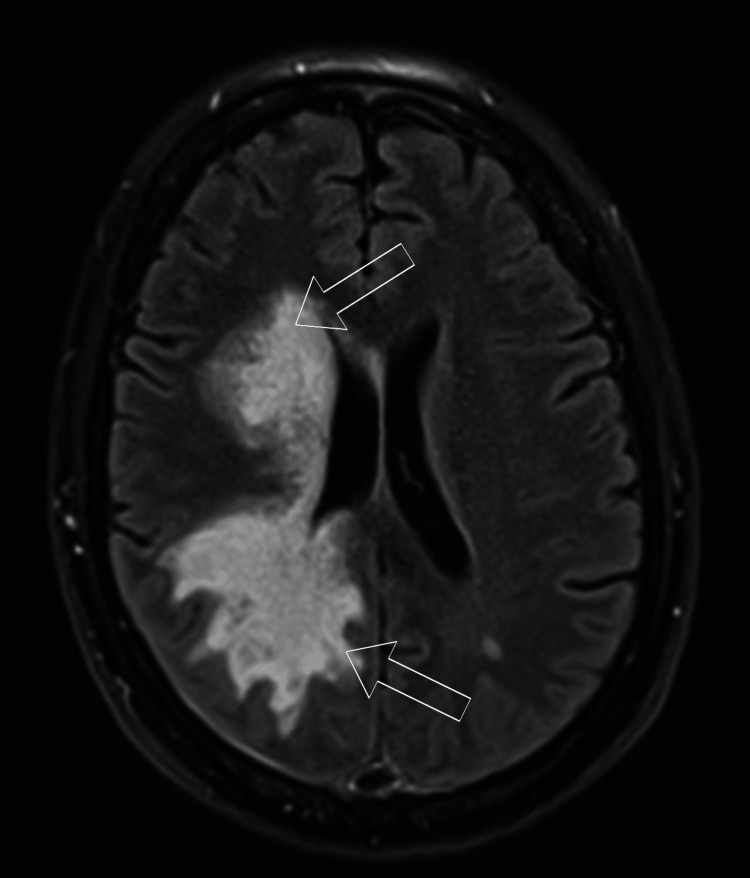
Follow-up post-treatment T2 FLAIR sequence T2 fluid-attenuated inversion recovery (FLAIR) sequence of repeat MR demonstrating a persistent signal in the associated areas as depicted by the unfilled arrows.
